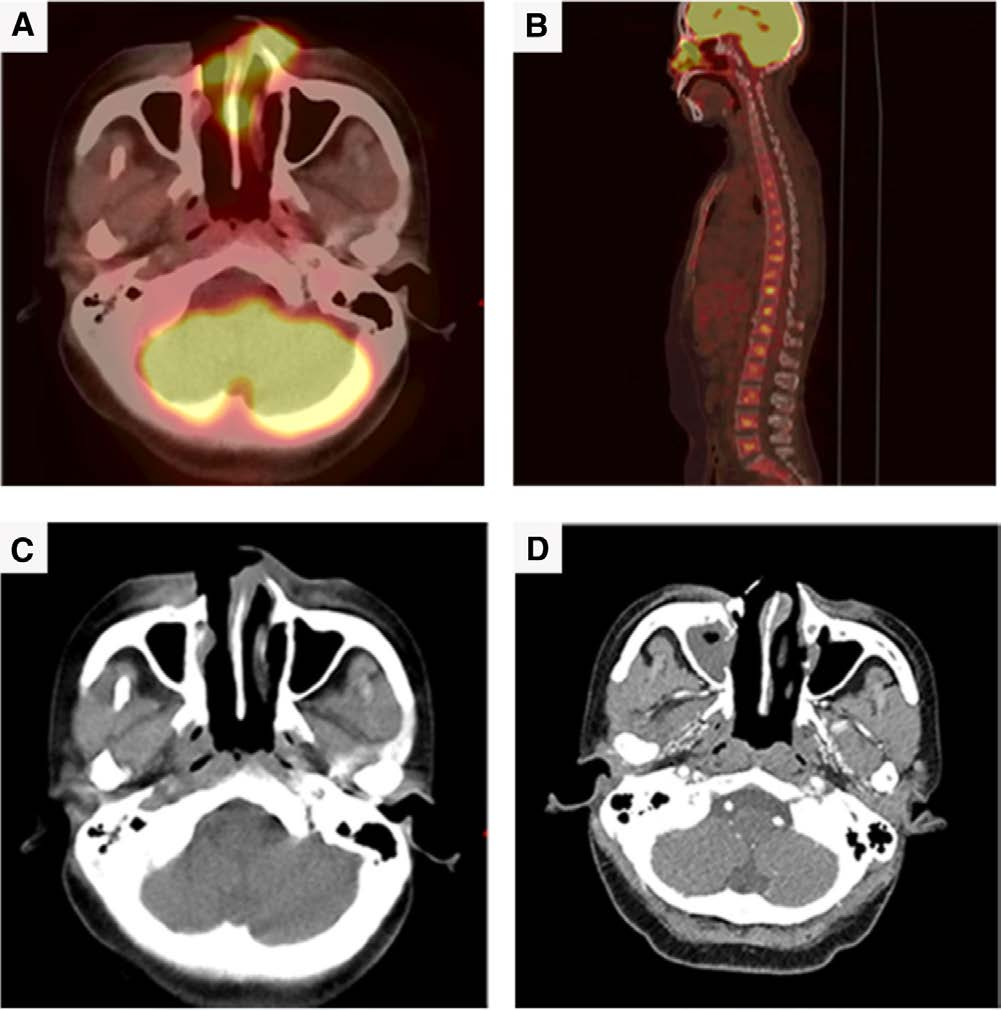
Images of case 1. (A) The maximum standardized glucose uptake value (SUVmax) of tissue in the right nasal, bilateral anterior and nasal septum was 4.8 on PET/CT scan after P-GEP treatment in May 2020. (B) The standardized glucose uptake value range was 2.0 to 4.8 in the spine in the sagittal section. (C) After the nasal biopsy, the thickened mass in the right nasal cavity, nasal septum and bilateral anterior nasal cavity on CT before immunotherapy. (D) After 2 cycles of immunotherapy, the swelling soft tissue was regressed in the bilateral nose adjacent to the face, the front part of the right nasal cavity and the septum subsided on enhanced CT in July 2020. PET/CT = positron emission tomography-computed tomography, SUVmax = the maximum standardized glucose uptake value.
